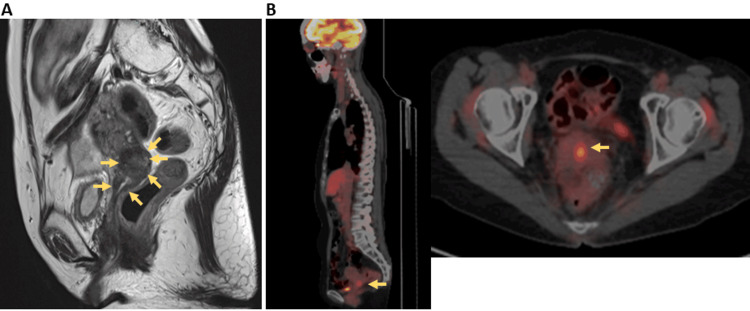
Imaging of recurrent cervical cancer. (A) Sagittal T2-weighted MRI. The yellow arrows indicates a cervical mass extending to the upper third of the vagina, suggesting recurrent cervical cancer. (B) FDG-PET/CT. The yellow arrow highlights increased FDG uptake in the cervix, consistent with solitary recurrent cervical cancer. FDG-PET/CT: 18F-fluorodeoxyglucose positron emission tomography/computed tomography
